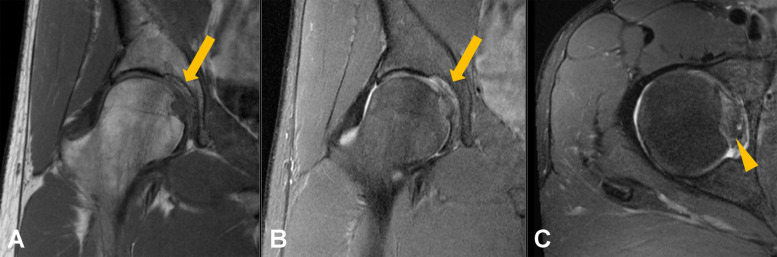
Representative coronal T1-weighted images (A), coronal fat-suppressed T2-weighted images (B), and transverse fat-suppressed T2-weighted images (C) of a 42-year-old man presenting with atraumatic right-sided hip pain for 8 months. Signal abnormalities of the pulvinar replacing more than 50% of the fat pad (grade 2) are highlighted by arrows in A and B. Note the concomitant signal abnormalities and thickening of the ligamentum teres (arrowhead, C)
